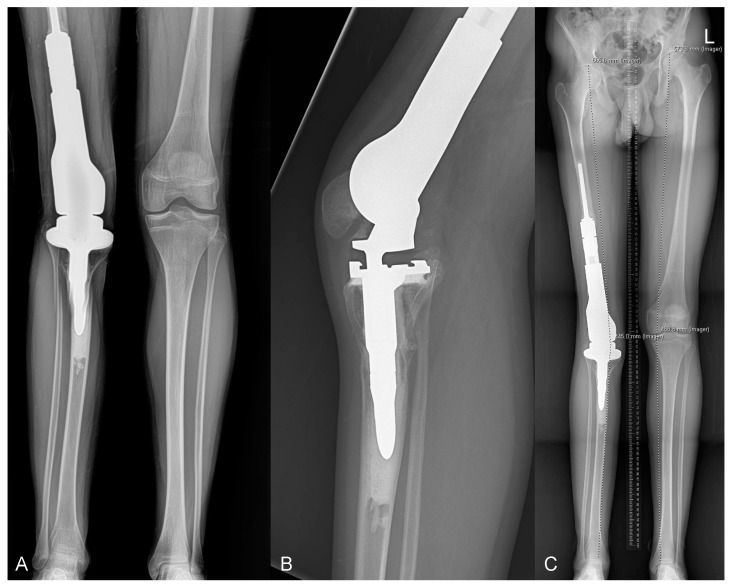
Imaging of a patient with history of high-grade osteosarcoma of the distal femur diagnosed at age 11 and treated with resection and reconstruction using Global Modular Replacement System (GMRS) extendable distal femur and hinged knee prosthesis. Patient underwent multiple standard lengthening procedures over 6 years (type 3A failures). (A) Leg length evaluation radiograph at age 15. Radiographs at age 16 showing (B) flexion contracture and arthrofibrosis and (C) leg-length discrepancy of 3.2 cm (type 6A failure), necessitating revision and eventual replacement with GMRS adult implant.
